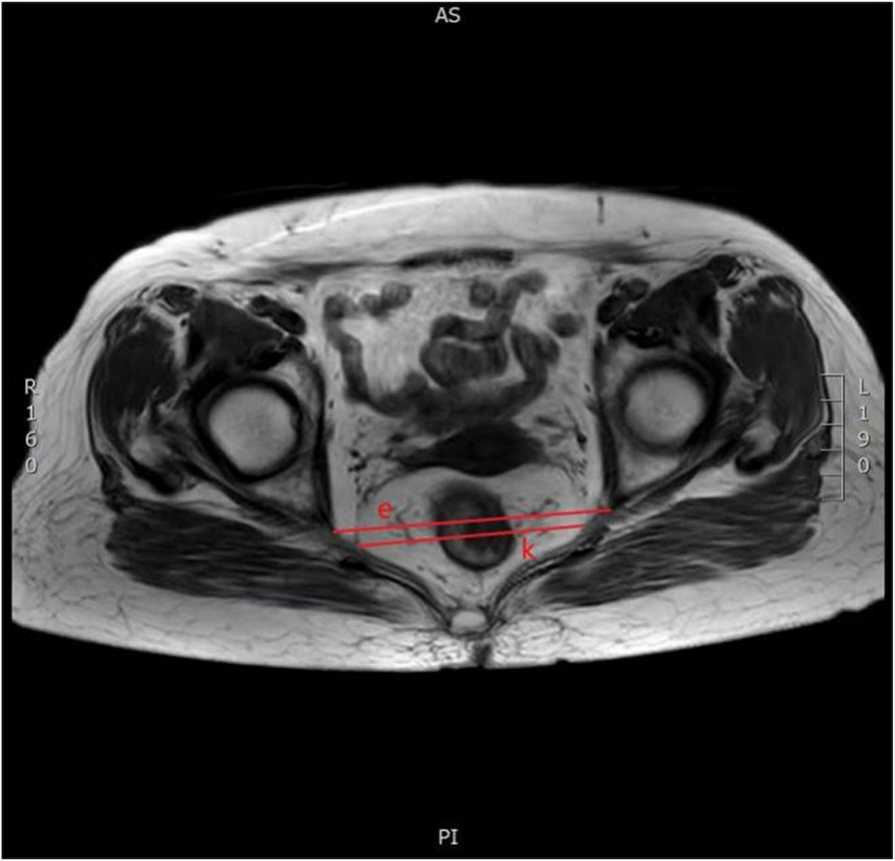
Horizontal pelvic magnetic resonance images: e pelvic outlet transverse diameter and k mesorectal fat area
